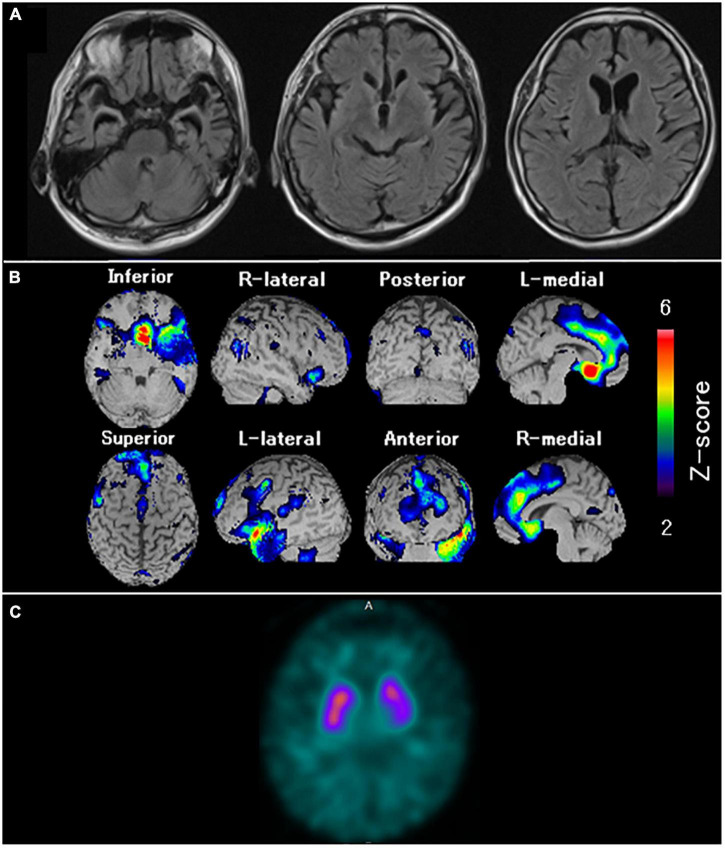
Neuroradiological findings in Case 6. (A) Magnetic resonance imaging shows marked bilateral anterior temporal lobe atrophy, predominant on the left side and the orbitofrontal cortex. (B) Brain perfusion single photon emission computed tomography (SPECT) easy Z-score Imaging System analysis (FUJIFILM Toyama Chemical Co., Ltd., Tokyo, Japan) shows significant hypoperfusion in the bilateral anterior temporal lobes, predominantly in the left side, the orbitofrontal cortex, and the medial frontal lobe. The color scale for the Z-score is shown on the right side of the figure. Presented in color if the Z-score was > 2. (C) Dopamine transporter (DAT) SPECT shows reduced DAT availability in the bilateral striatum, predominant on the left side.
